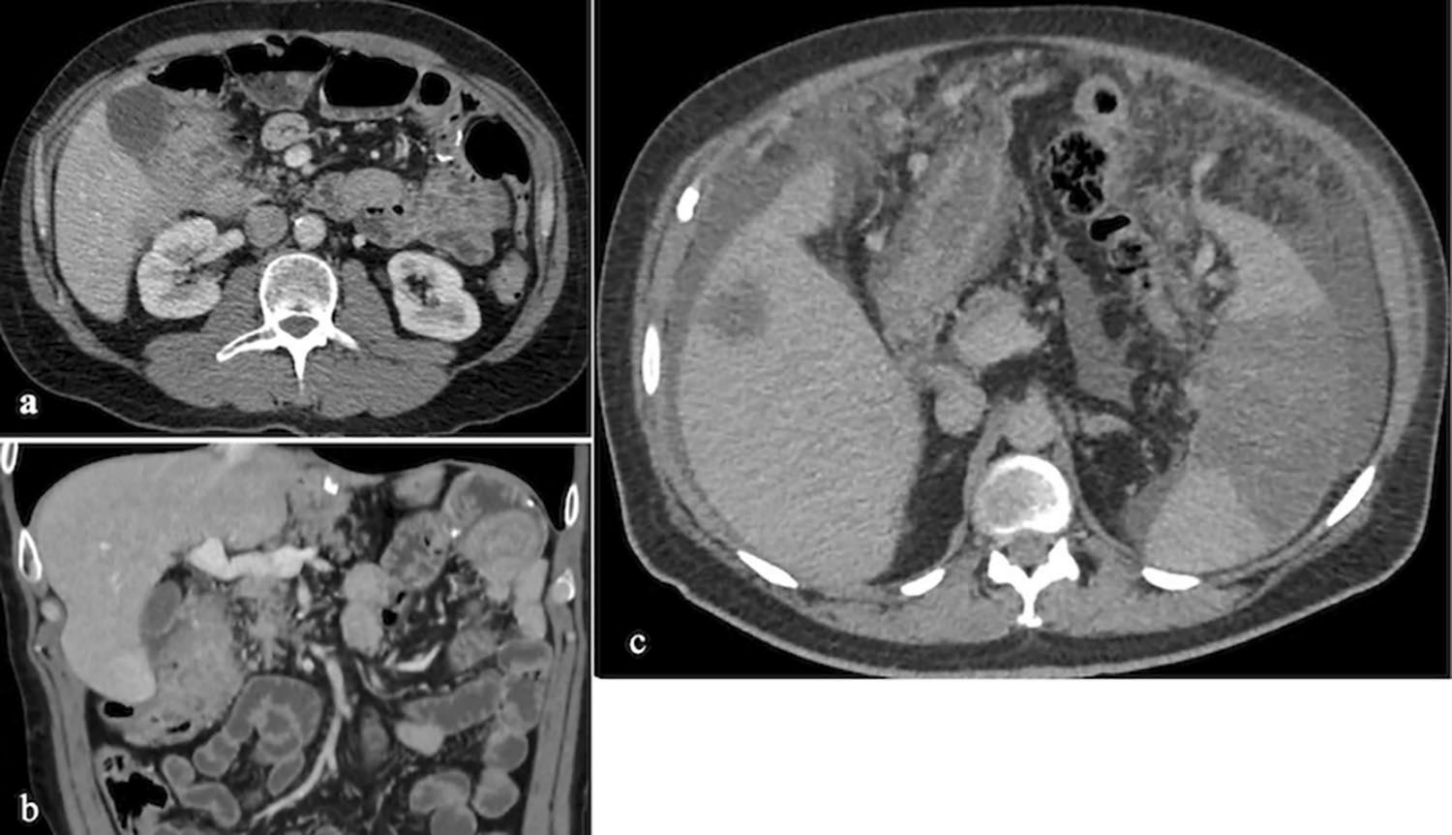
IV contrast enhanced a. Axial and b. Coronal CT images of a 48-year-old male patient with duodenal metastasis of gastric tumor located at cardia. There is an irregular solid mass with necrotic changes and heterogenous enhancement. c. IV contrast enhanced axial CT image of a 52-year-old female patient with colon carcinoma and peritoneal metastasis. In the second—(D2) segment of duodenum there is irregular wall thickening, wall enhancement and paraduodenal fluid collection. Additionally, there are peritoneal nodular lesions, mental heterogeneity and metastatic mass at the fifth segment of liver
